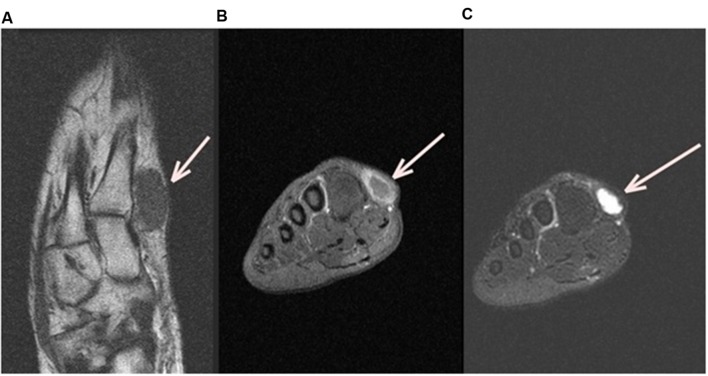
Left Foot MRI (Magnetic Resonance Image). Axial MRI T1 left foot image (A) shows a subcutaneous ovoid mass of 3cm × 2cm in contact with the first metatarse (white arrow), coronal T1 fat sat with gadolinium (B) and Coronal T2 fat sat (C) show, respectively, an hyposignal T1 and a hyper signal T2 with peripheric enhancement after injection.
